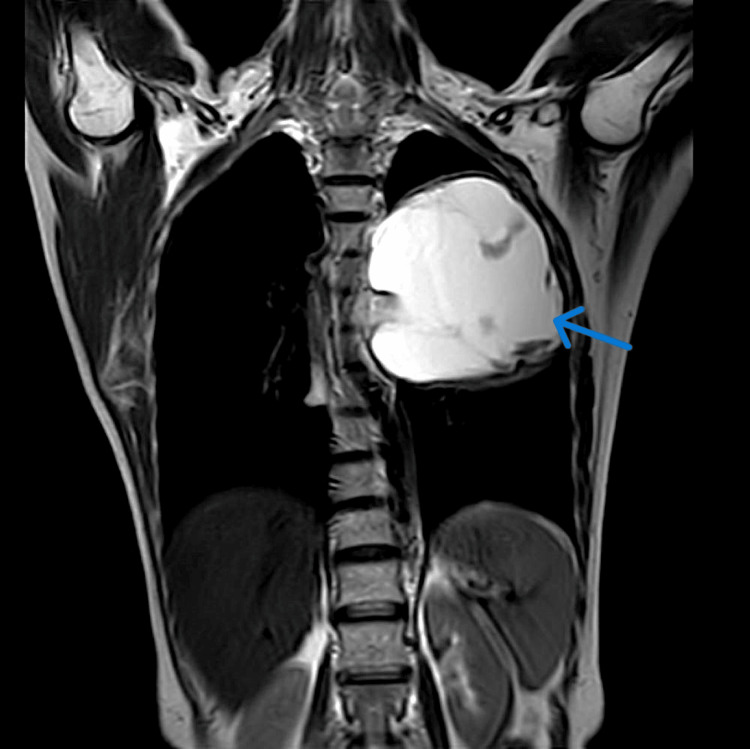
MRI image showing evidence of a well-defined T2 hyperintense lesion (blue arrow) in the upper lobe of the left lung.
